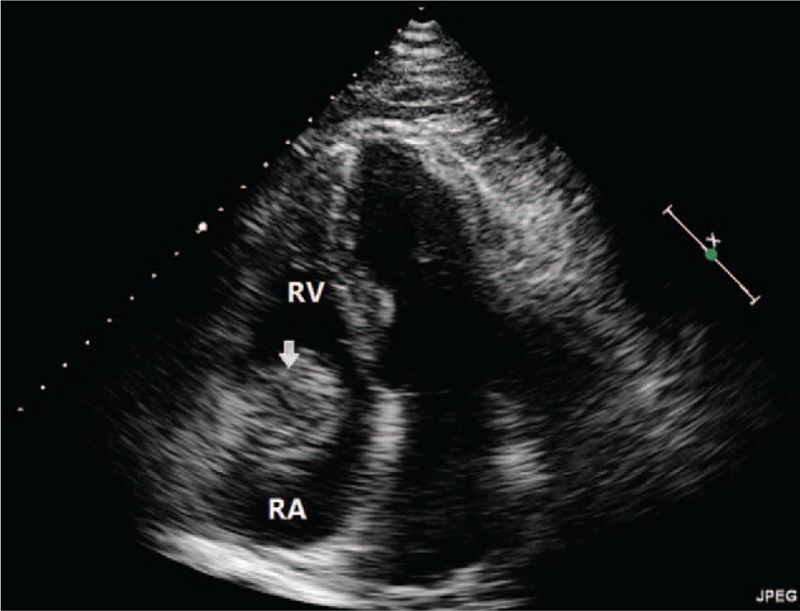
Transthoracic echocardiography revealed a 37 × 37 mm^2^ round like acoustic shadow above the tricuspid valve ring and showed no swing in the chambers of the heart. The internal echogenicity was medium mixed with hypoechoic signal with a strong echo capsule and a clear boundary.
